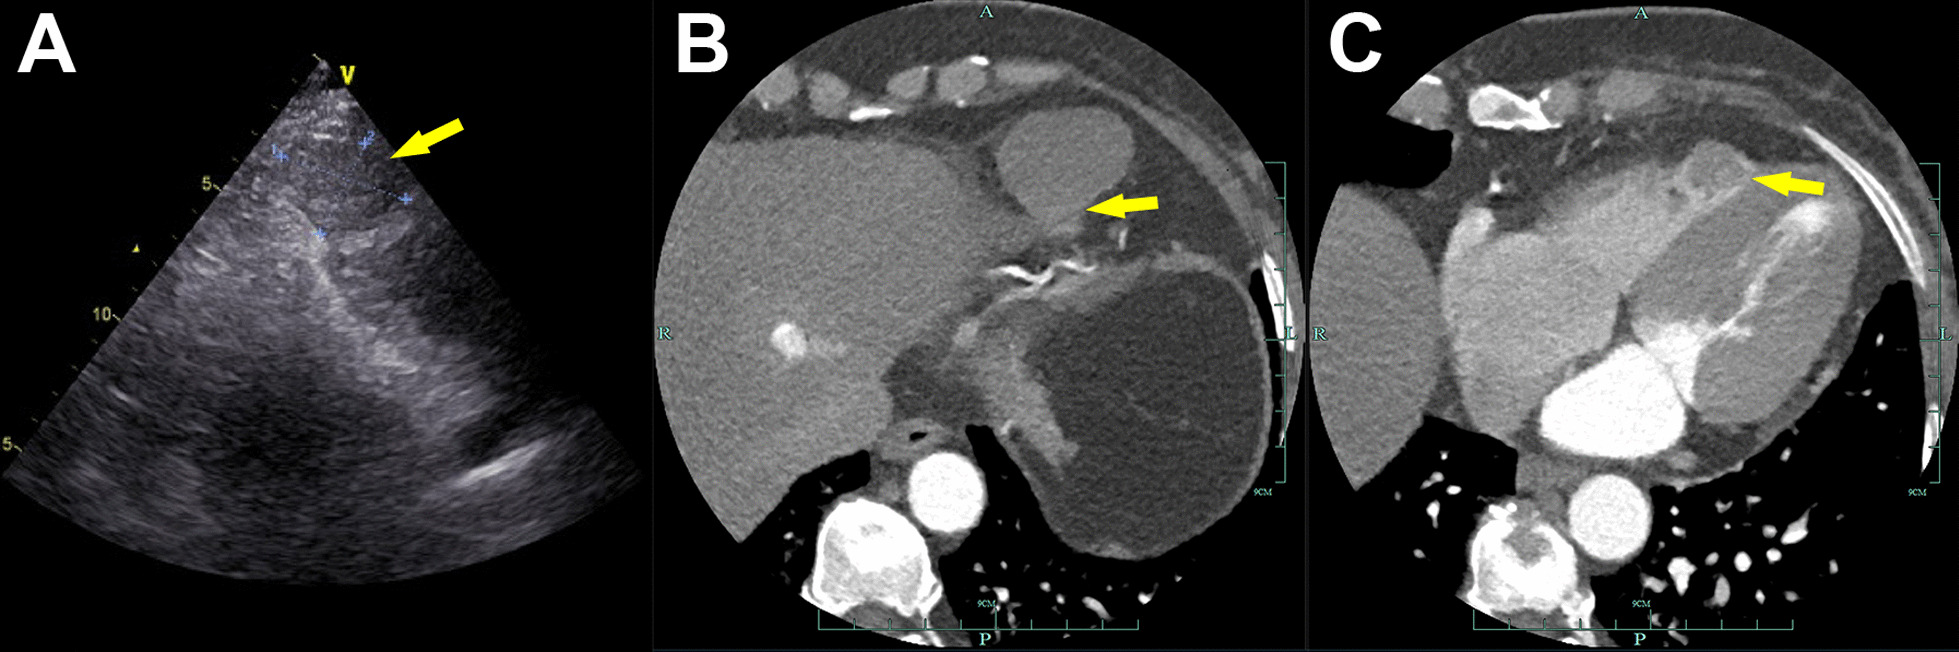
Preoperative imaging. a Re-transthoracic echocardiography showed the size of the mass as 4.0 × 3.0 cm(arrow); b Coronary CTA showed the mass has a clear boundary with the RV(arrow), but c showed an infiltrating mass appearing to be connected to the RV simultaneously (arrow)
